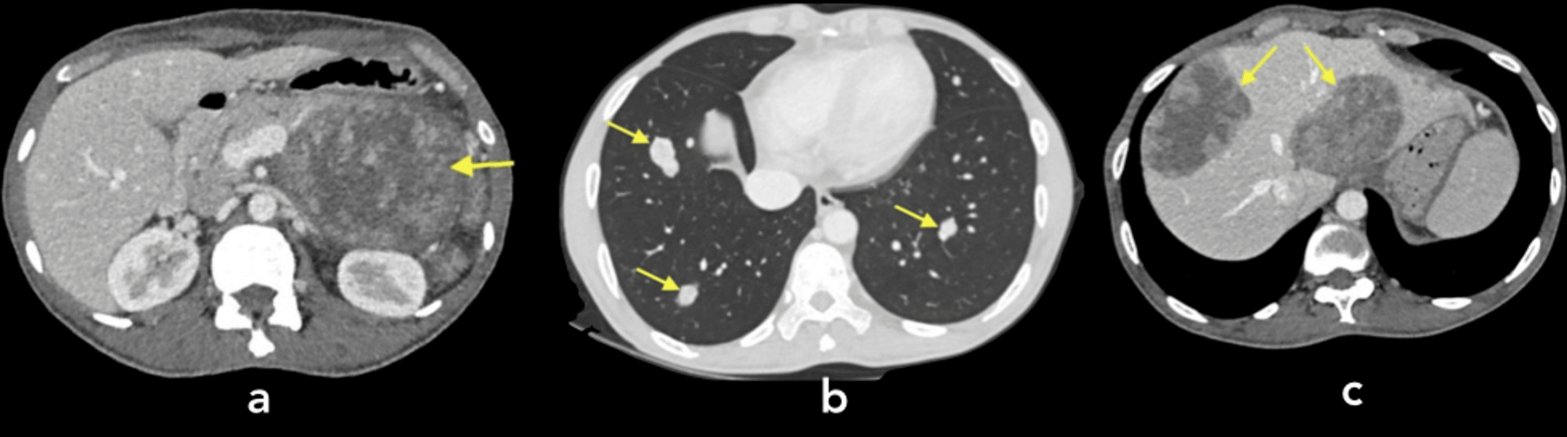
CT of the abdomen with contrast. Contrast-enhanced CT scan of the abdomen (a) showing a large heterogeneous mass in the pancreas, measuring approximately 10 × 9 × 12 cm, with evidence of necrotic foci (yellow arrow). The mass can be seen displacing adjacent structures, including the ileum, transverse, and descending colon, and causing a mild mass effect on the left kidney. Multiple metastatic nodules (yellow arrows) are also visible in both lungs (b). Metastatic lesions are present in the liver (c), with the largest lesion in segment II measuring 8 × 8 cm (yellow arrows).
